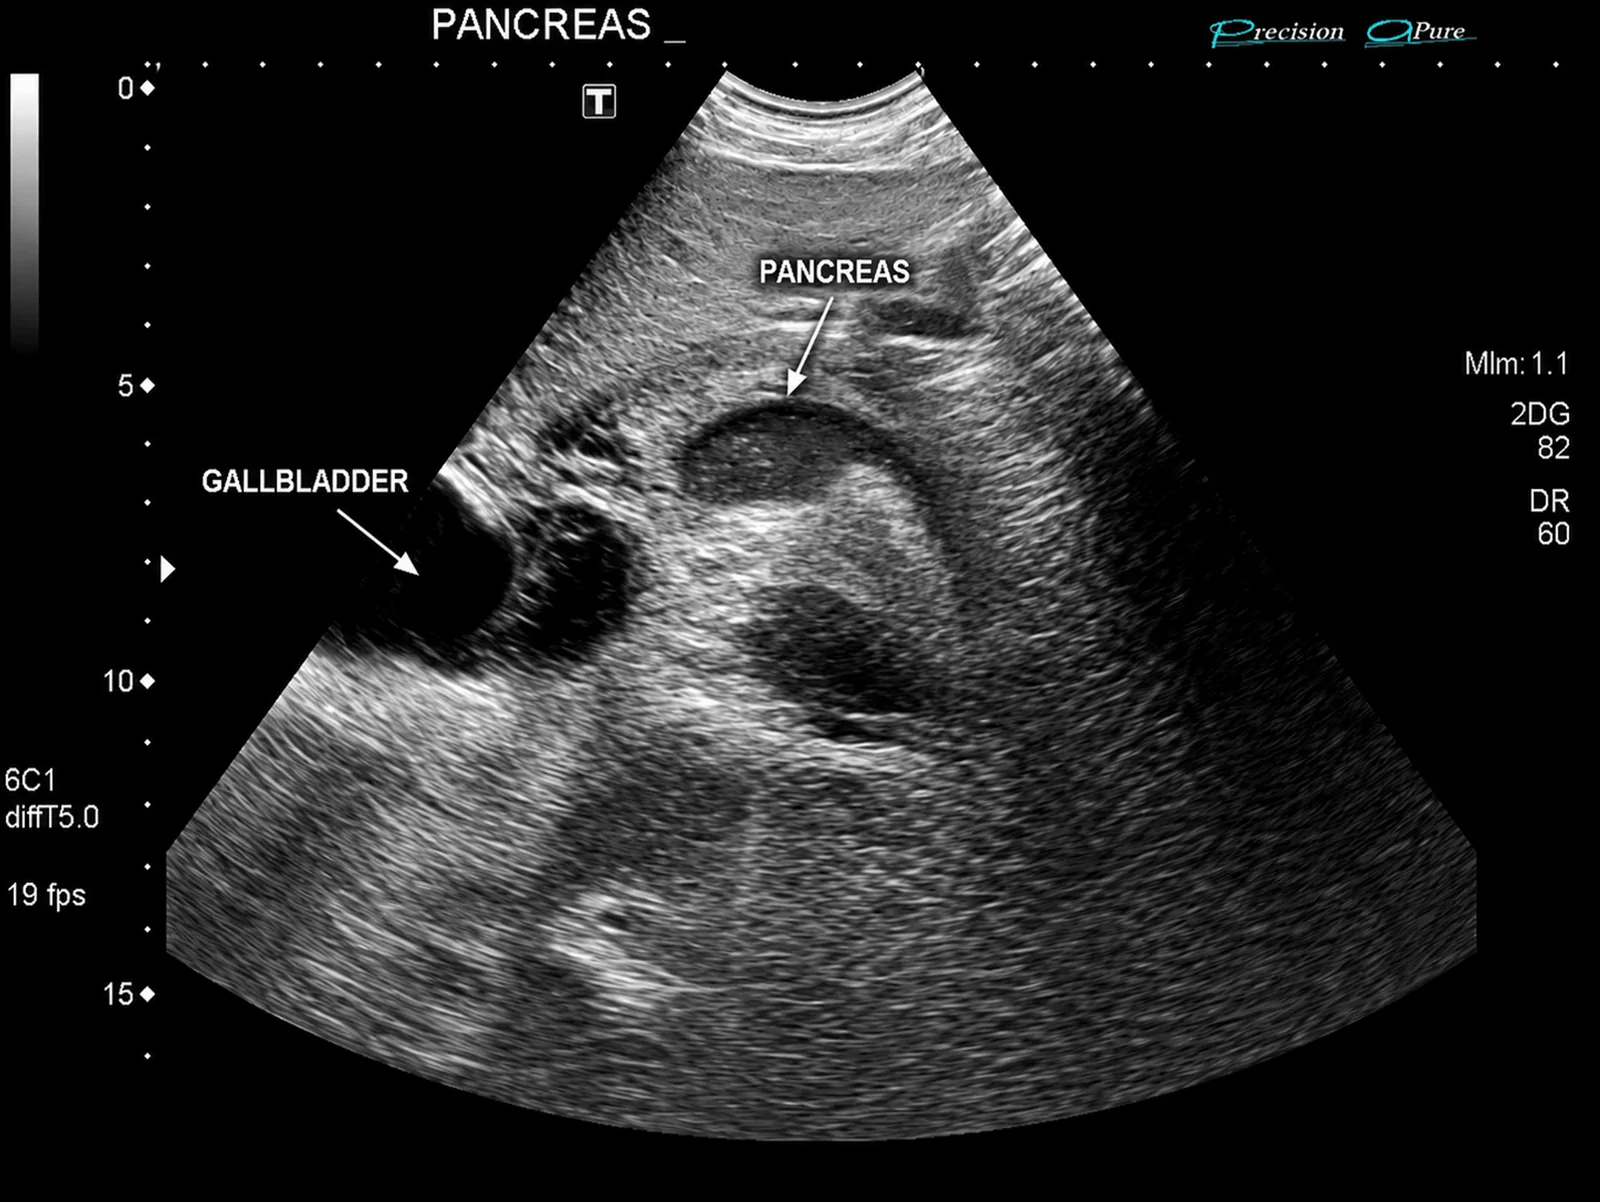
Right upper quadrant abdominal ultrasound Gallbladder sludge and borderline gallbladder wall thickening can be seen without frank sonographic evidence of acute cholecystitis, pericholecystic fluid, or biliary ductal dilatation.
